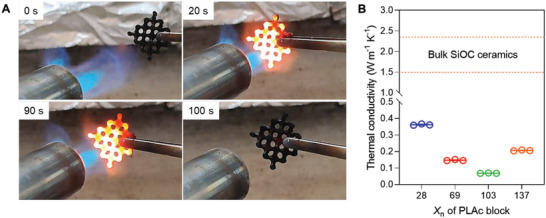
Thermal properties of PIMS ceramics. A) Optical images of 3D printed PIMS ceramic lattice demonstrating thermal shock resistance during heating in a butane gas flame (*T* > 1200 °C). The gas flame was turned off after 90 s. B) Thermal conductivity values at 400°C for PIMS SiOC ceramics versus PLAc‐CTA *X*
